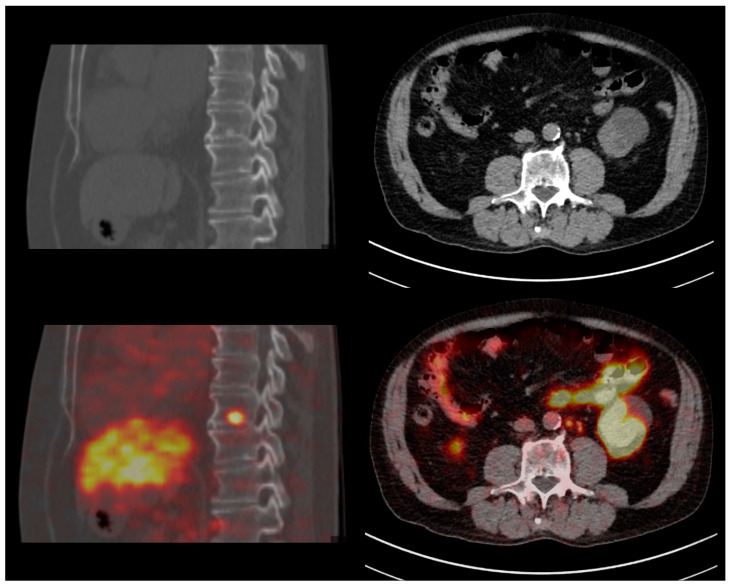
Clinical cases—Restaging of two early-recurrent prostate cancer patients with digital PSMA-PET/CT. Left column: 73 yo patient with Gleason 9 (4 + 5) pT3bN1 prostate cancer, treated with radical prostatectomy + linfadenectomy (03/2017) and salvage RT (07/2017); biochemical recurrence with PSA 0.23 ng/mL. PSMA-PET/CT identified a vertebral (D9) osteoblastic metastasis with intense tracer uptake. Right column: 75 yo patient with Gleason 9 (4 + 5) pT3bN1 prostate cancer, treated with radical prostatectomy and linfadenectomy (01/2019); biochemical recurrence with PSA 0.22 ng/mL. PSMA-PET/CT identified multiple extra-pelvic (para-aortic) subcentimeter nodal metastases (maximum diameter: 5 mm).
